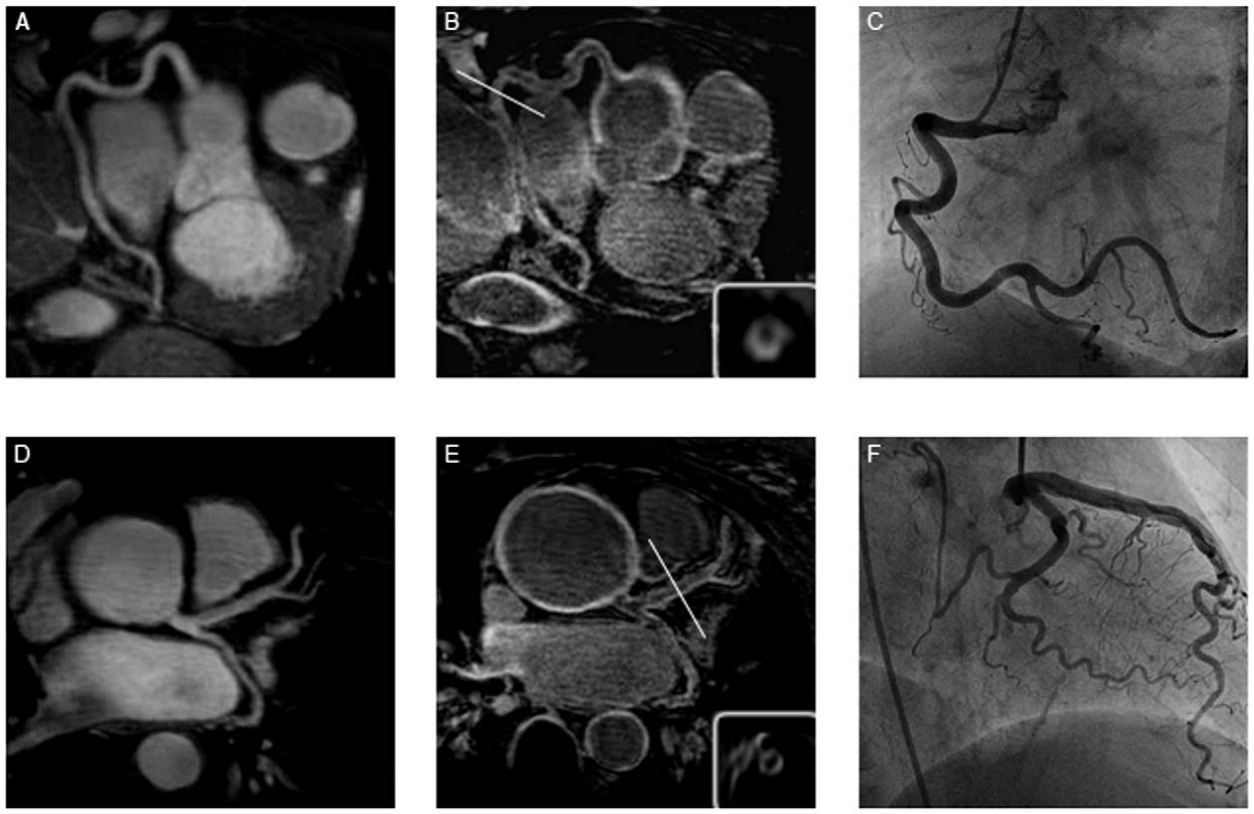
Representative T2prep images of the right coronary artery (RCA) (A) and the left main stem (D) in a patient with TA. B and E show the post IR scan of the same patient, revealing late gadolinium enhancement (LGE) of the coronary artery wall. C and F depict the corresponding x-ray angiography.
